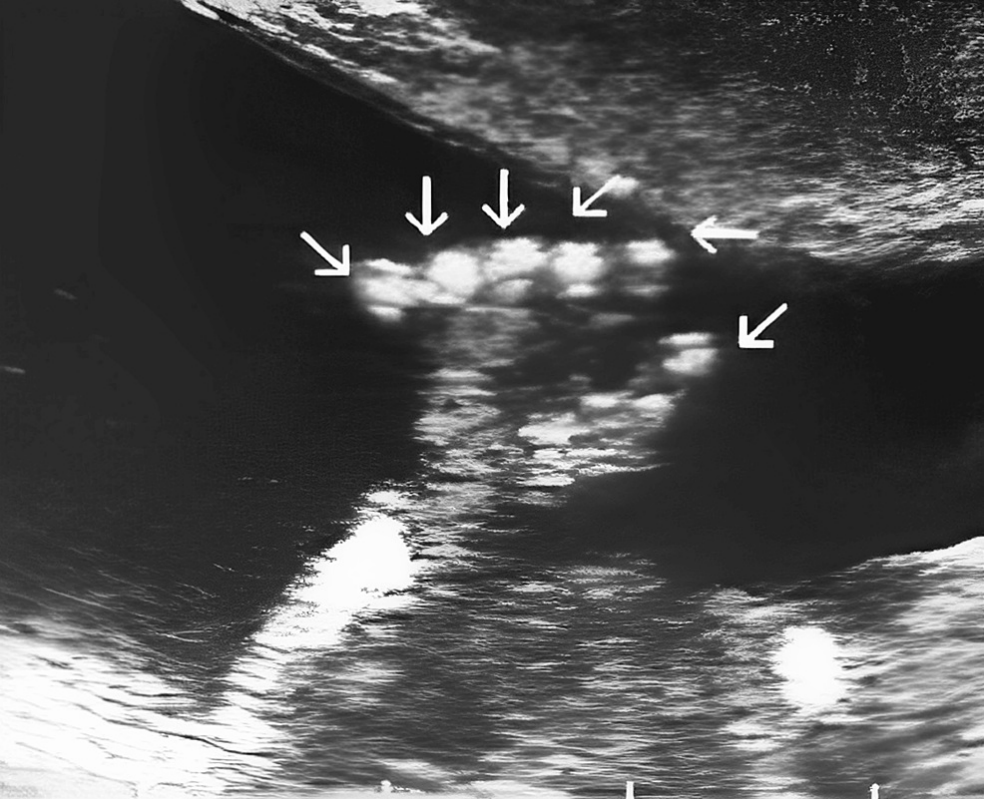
Level II ultrasound scan showing polydactyly.
